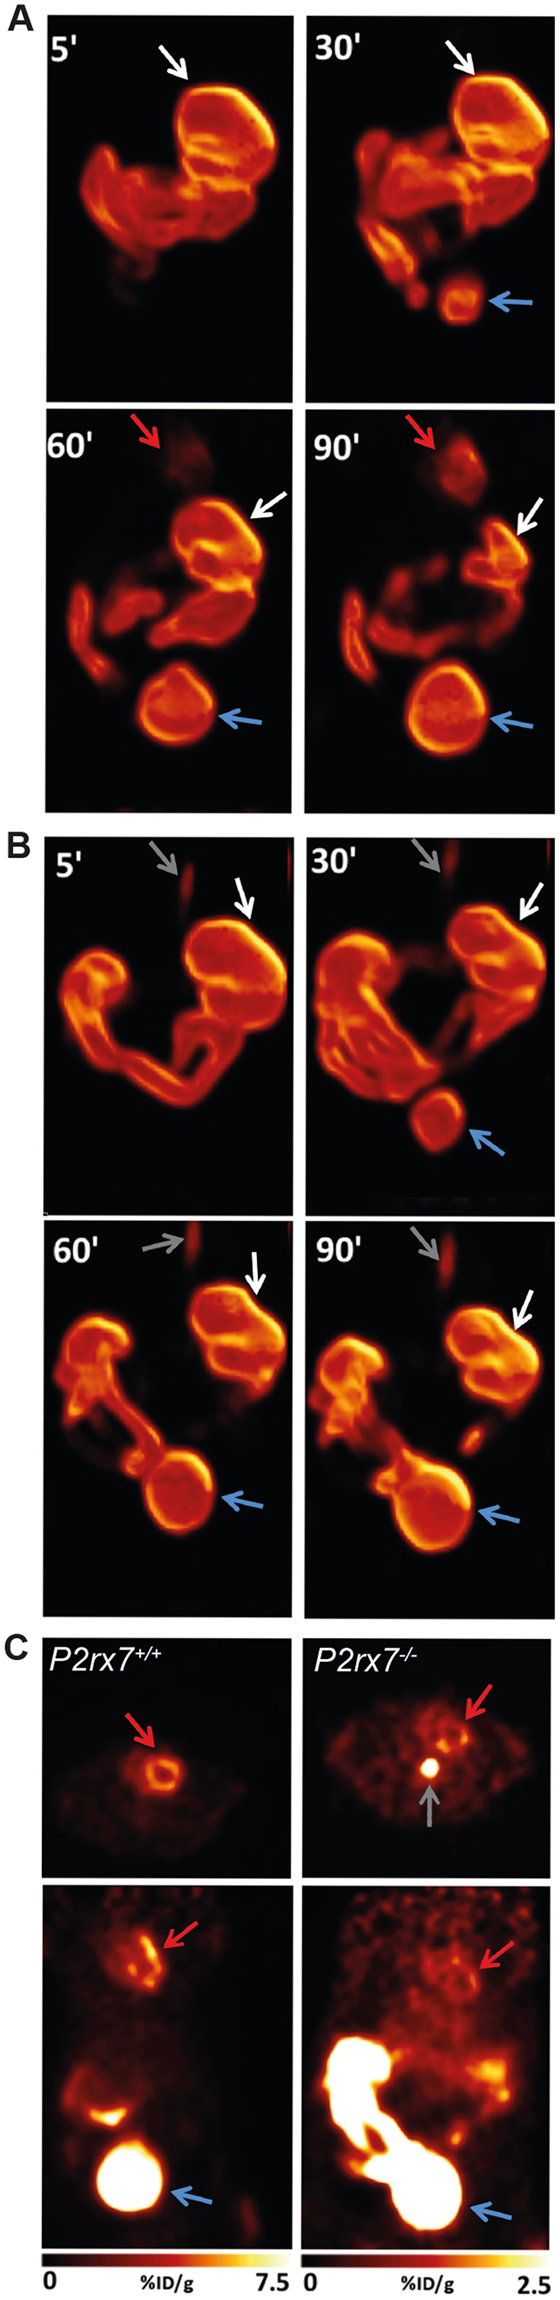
Maximum intensity projection 3D rendering at different time points following *per os* inoculation of FDG in the stomach of (A) *P2rx7*
